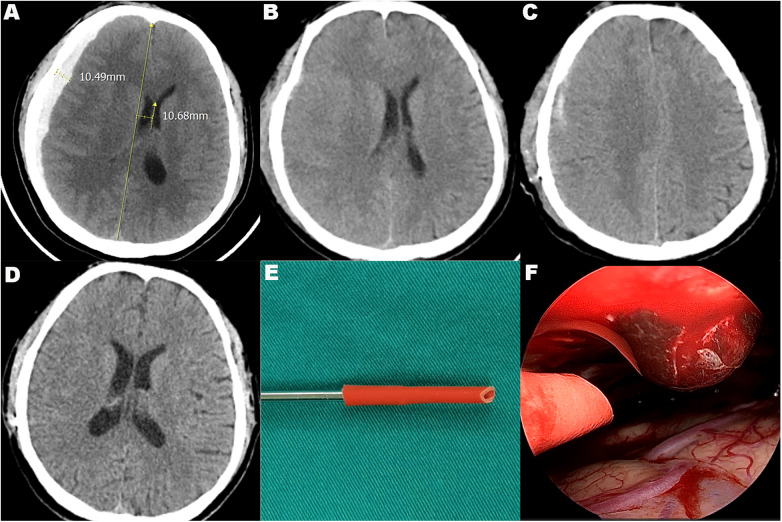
(A) Head CT scan at admission depicting an acute subdural hematoma and pronounced midline shift. (B,C) CT on postoperative day 4 showed sufficient hematoma removal and residual hematoma caused by venous bleeding intraoperatively. (D) The midline shift improved 46 days after surgery. (E,F) The rubber tip connected to the suction cannula was clipped into a slope shape to prevent brain damage.
